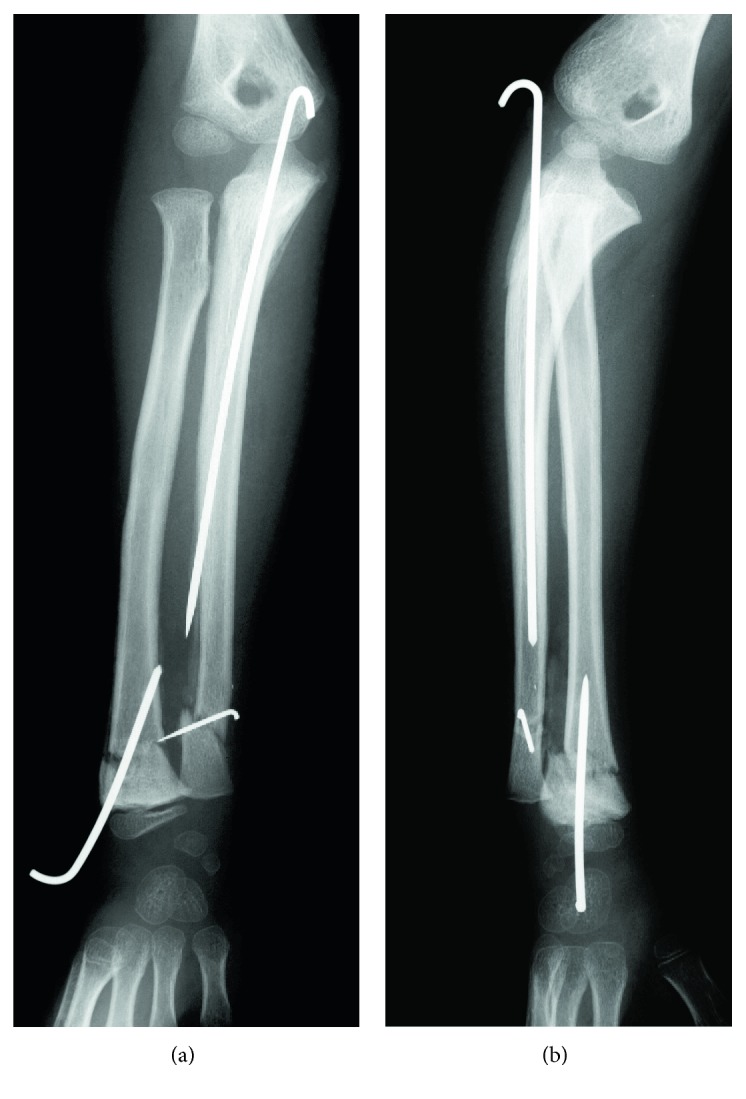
X-rays postoperation. (a) AP view. (b) Lateral view.
